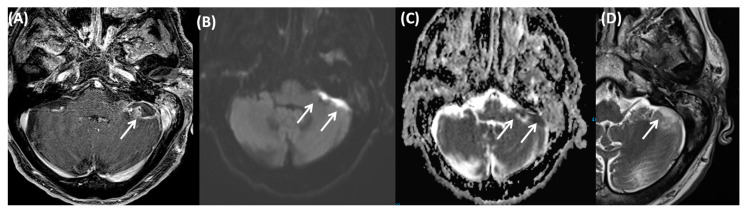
An 82-year-old male patient with intracranial infratentorial abscess after otomastoiditis. The abscess can be identified on T1-weighted fat-saturated sequences after contrast agent application (A), and can present with local signs of a meningeal irritation and demarked diffusion restriction (B,C). Axial T2-weighted imaging (D) shows intermediate intensity of the abscess.
